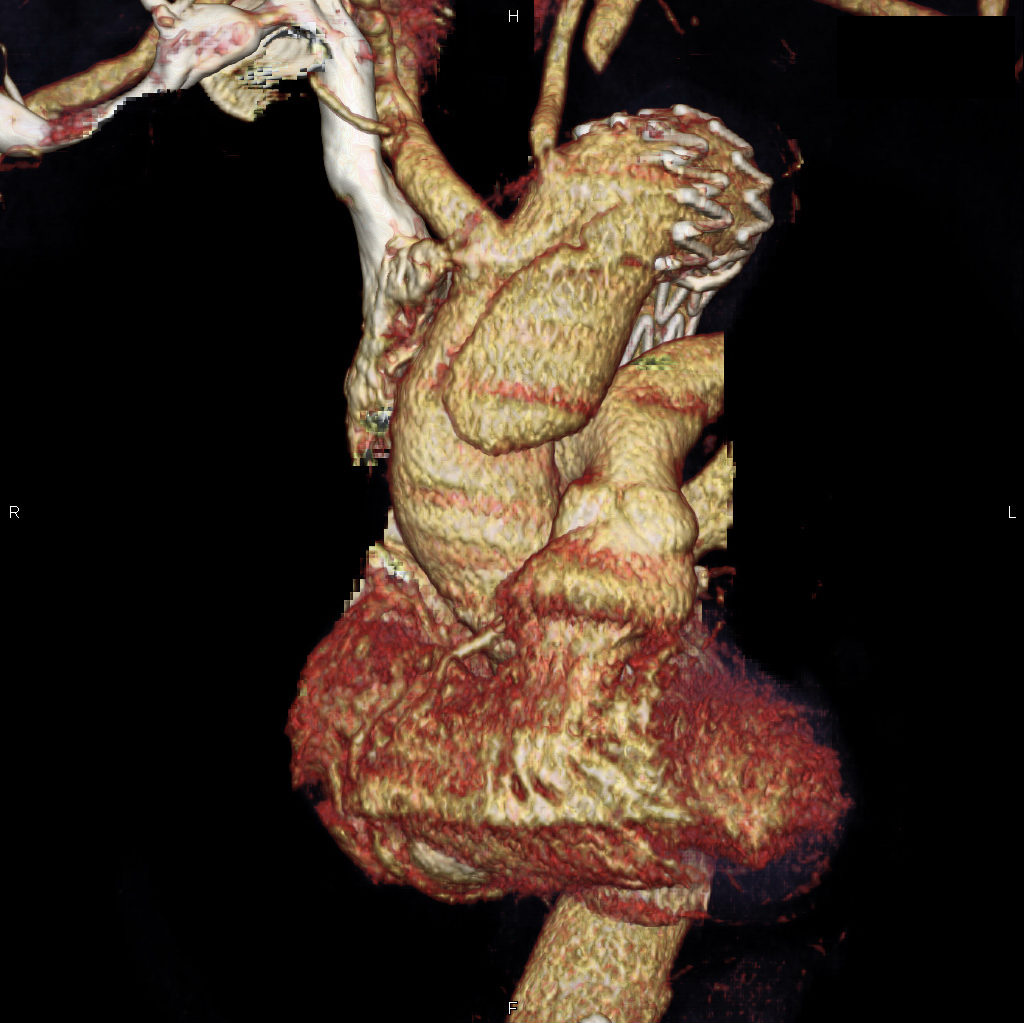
Three-dimensional computed tomographic reconstruction demonstrating the retro-A dissection.
